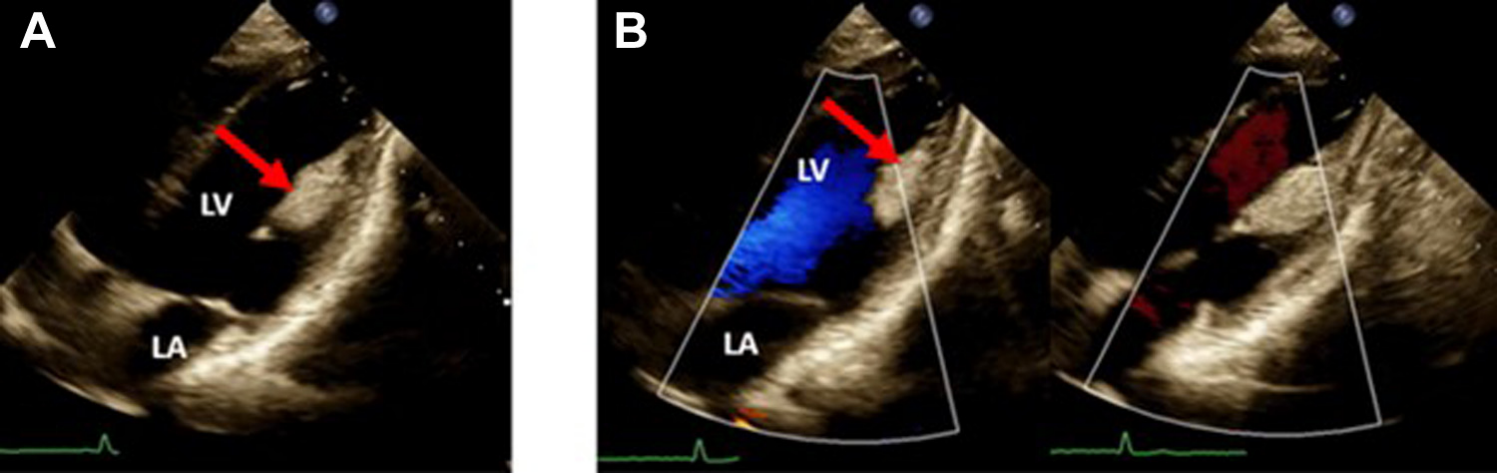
Two-dimensional TTE, rotated apical 4-chamber view, systolic phase without (A) and both systolic/diastolic phases with (B) color flow Doppler, demonstrates the echo-bright, posteromedial papillary muscle lipoma *(arrows)* without blood flow signal within the mass. *LA*, Left atrium; *LV*, left ventricle.
